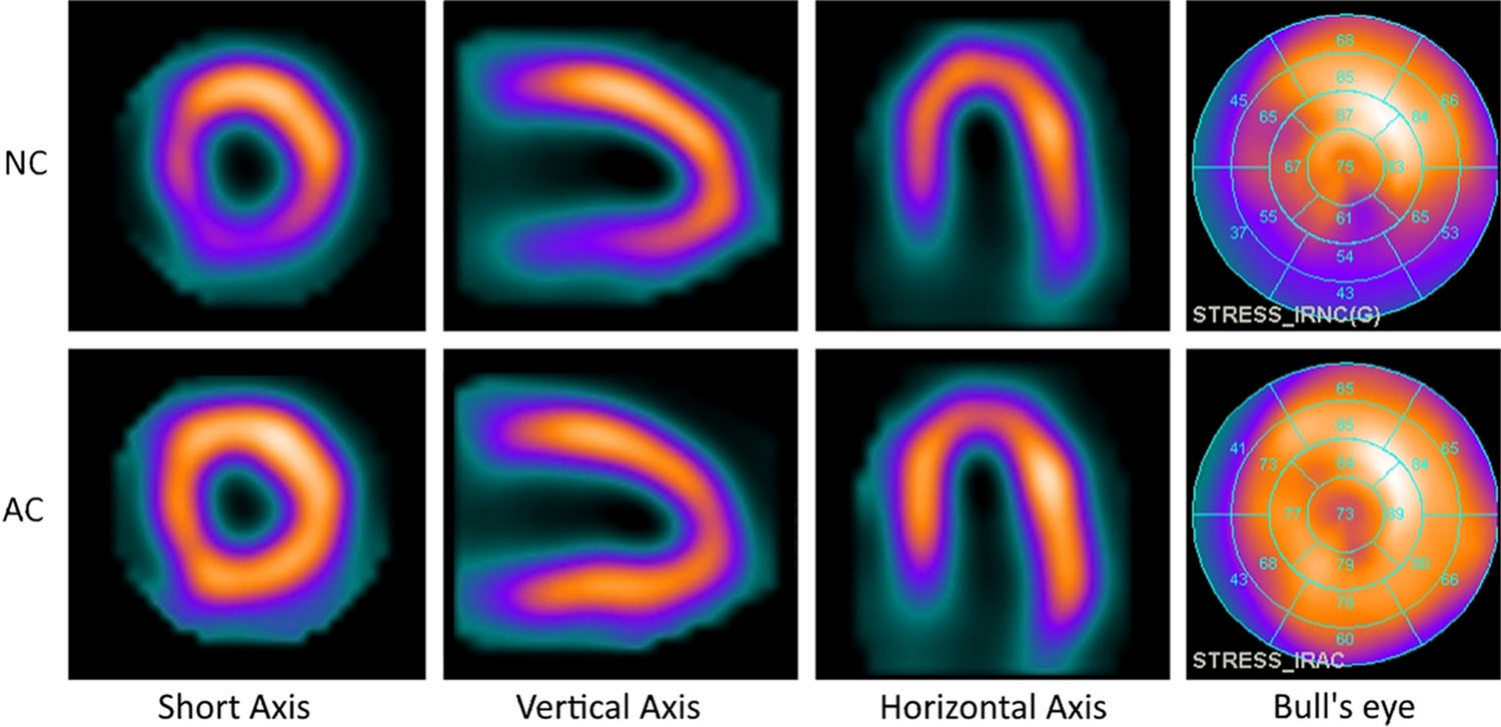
Key images from a stress MPI CZT-SPECT scan reconstructed without (*top row*) and with attenuation correction (*bottom row*), where a possible inferior defect in the non-corrected images is corrected using attenuation correction. The images are from a typical patient (72 year-old male, 67 kg, BMI 22 kg/m^2^). Corresponding short, vertical, horizontal axis and a parametric bull’s eye plot are shown from left to right
